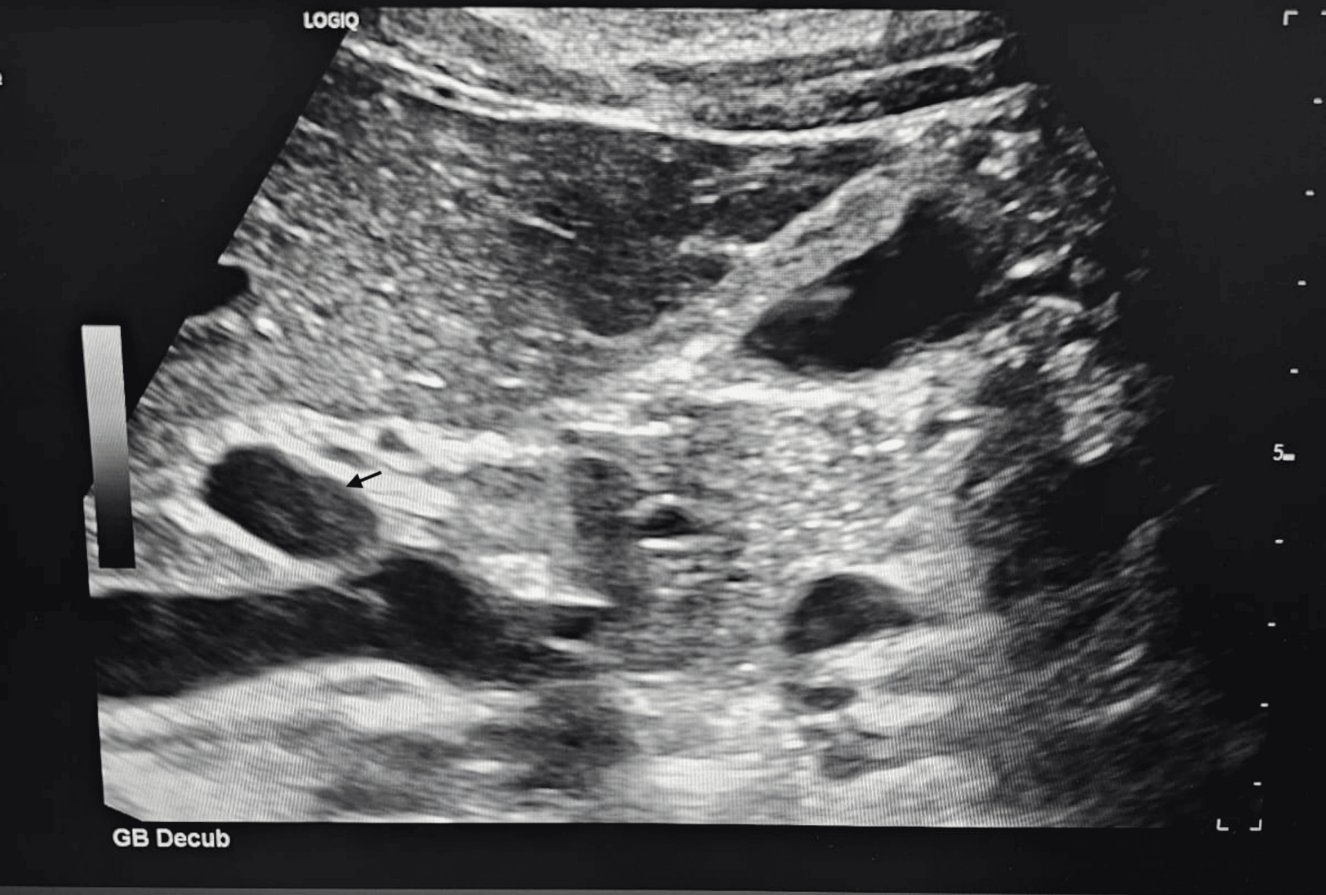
Abdominal ultrasound showing gallbladder wall edema with minimal intraperitoneal free fluid. Abdominal ultrasound image depicting minimal free intraperitoneal fluid and diffuse gallbladder wall thickening with edema. Small gallbladder polyps measuring up to 2.8 mm were also visualized. The liver and spleen appeared normal in size.
